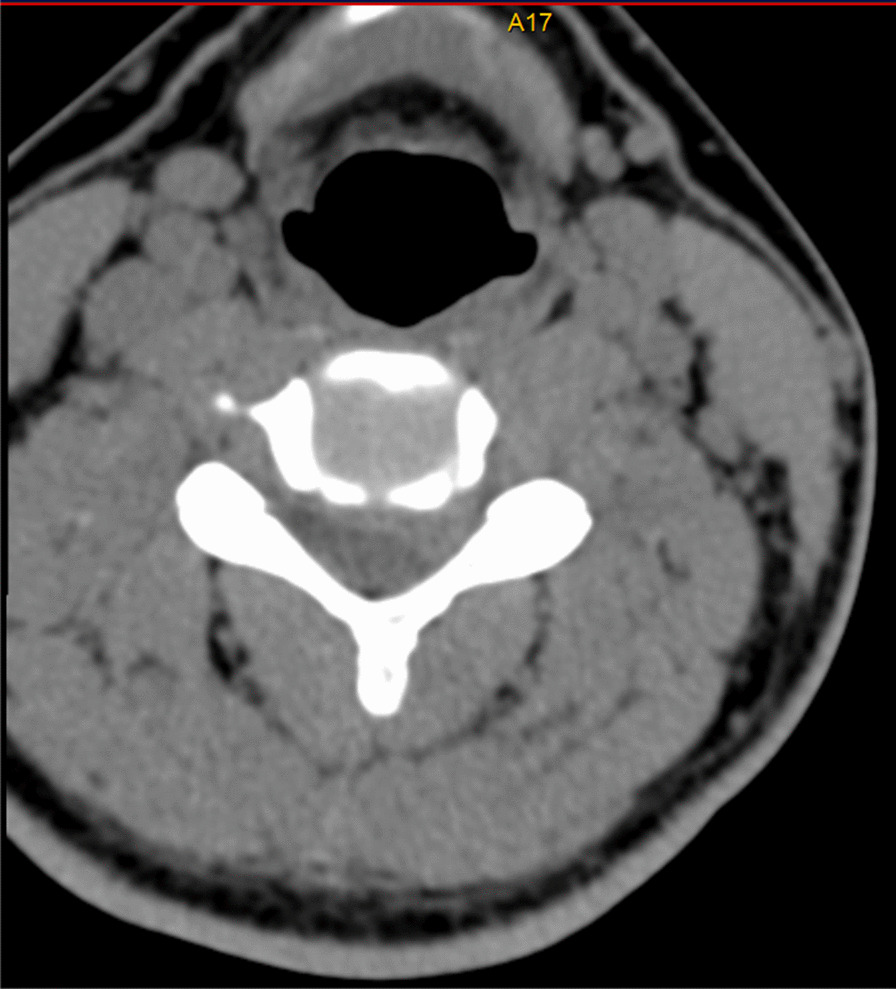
Preoperative CT showed giant cervical disc herniation
